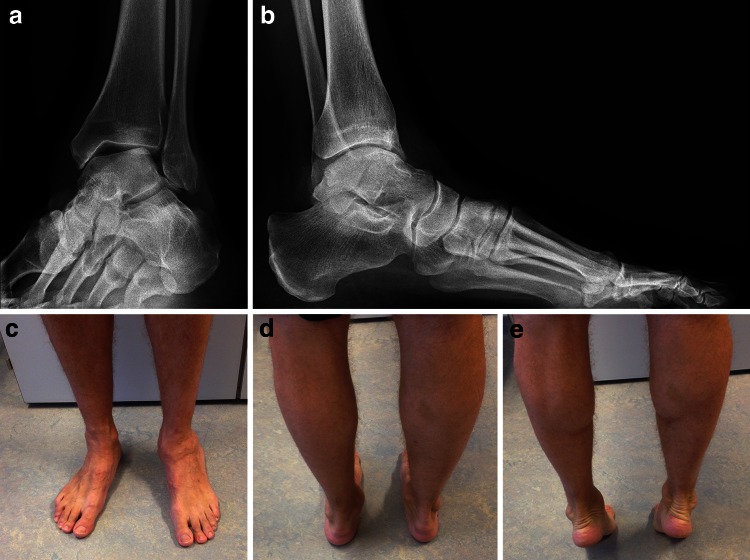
Chronic medial ligament instability. Radiographical (a, b) and clinical (c–e) distinct medial instability with talar tilt and hindfoot valgus of the *left foot*. The lateral X-ray (b) shows an intact talonavicular joint with the talar head still in an articulating position, suggesting that the spring ligament is intact. While standing on tiptoe (e), hindfoot valgus disappears due to the intact and functioning posterior tibial muscle
